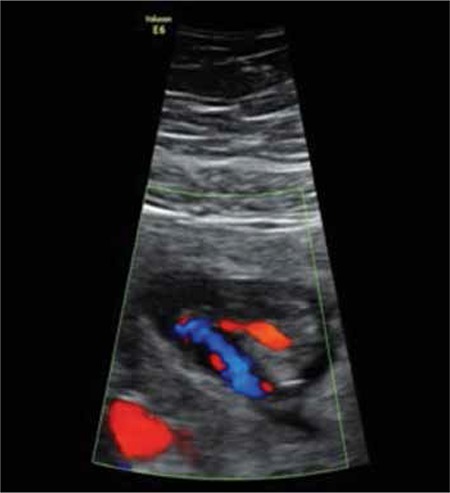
Color doppler ultrasonographic image shows single umblical artery
